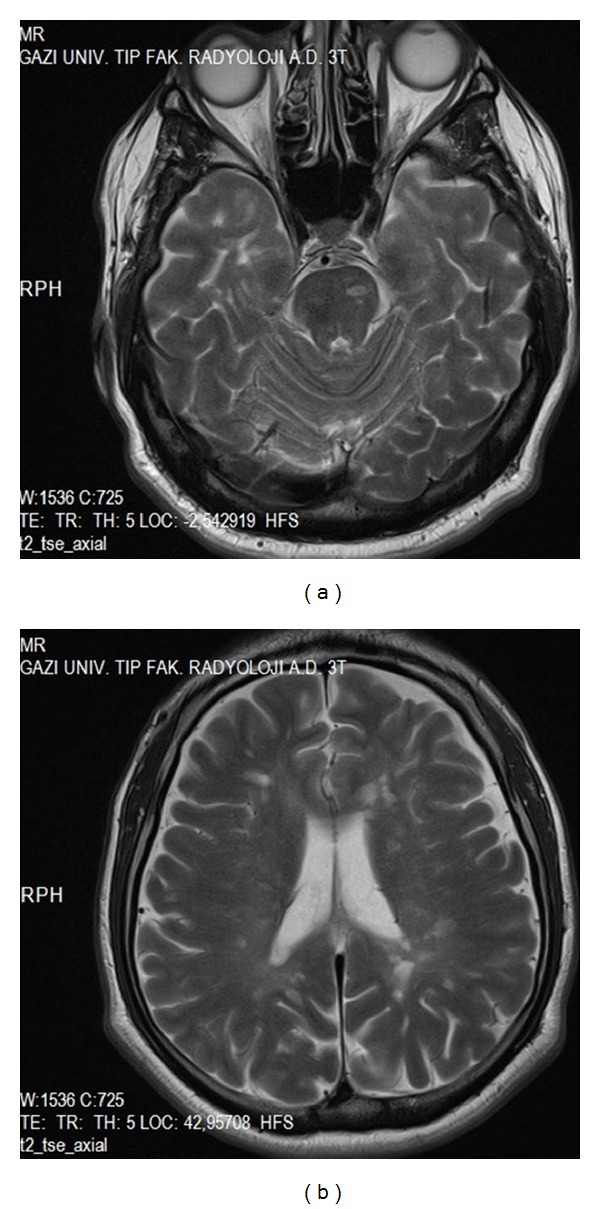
(a) Demyelinating periventricular lesions, (b) demyelinating lesions in the pons, T2 weighted axial section, and brain MRI.
